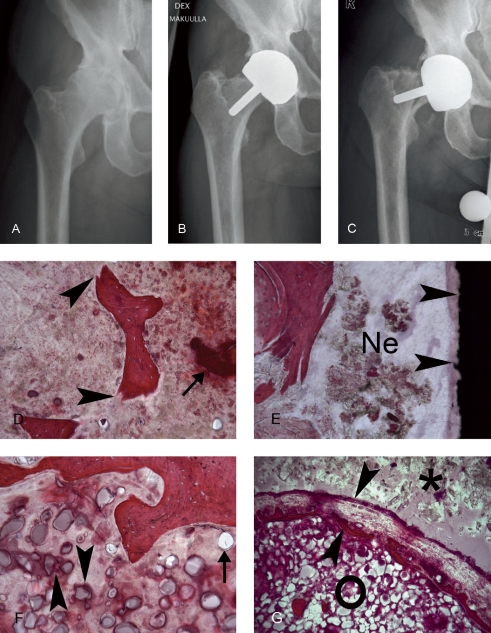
Radiographs of the right hip of case 1, a male patient with end-stage osteoarthritis. A. A preoperative anteroposterior radiograph showing good bone quality. B. A postoperative anteroposterior radiograph showing that the BHR implant is well-positioned. C. 46 months postoperatively, this patient fell on stairs. Radiographs showed a femoral fracture. D. A fractured bone trabecula (arrowheads) in an area adjoining necrotic bone (arrow). E. Implant-host tissue interface with some necrotic tissue (Ne) close to the black implant surface (arrows). F. Trabecular bone with marrow space, which contains phagocytozed (arrowheads) and extracellular (arrow) cement particles. G. Such cement particles (o) were often seen close to the host-cement interface (*), which in this section is already separated by an implant capsule (arrowheads).
